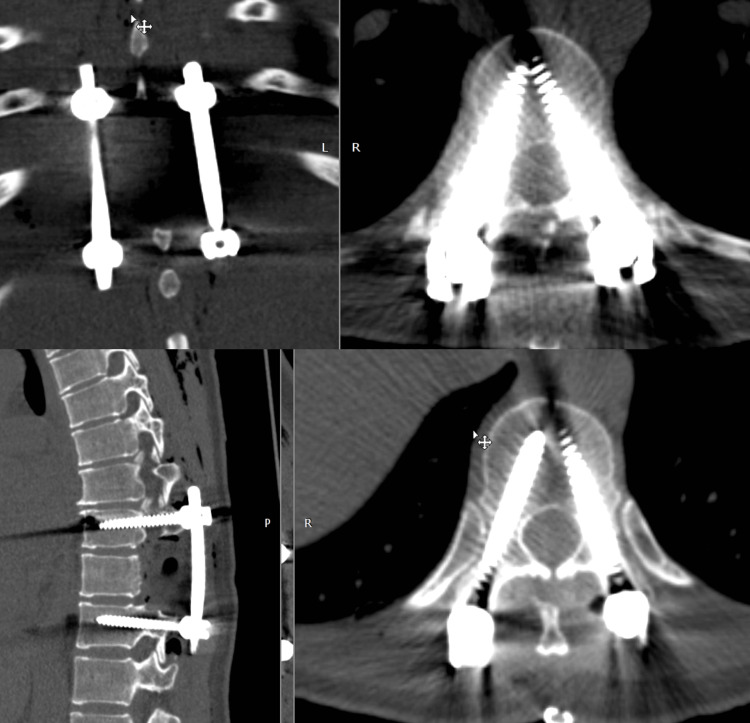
Postoperative CT of the thoracic spine demonstrating resection of the T10 lesion and posterior spinal stabilization.
